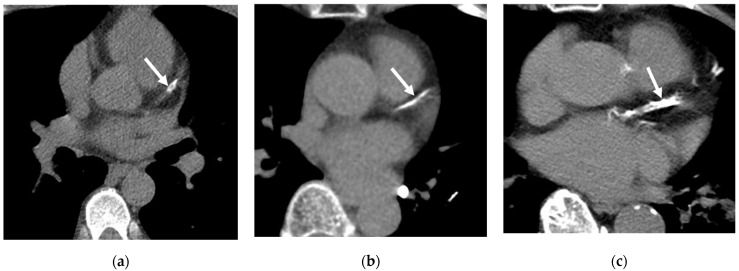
Qualitative assessment of coronary artery calcification (CAC) by low-dose computed tomography (LDCT). Axial LDCT scans demonstrate varying degrees of CAC. (a) Mild CAC with isolated flecks of calcification (arrow) in left anterior descending artery (LAD). (b) Moderate CAC with more extensive calcification (arrow) involving two segments of LAD. (c) Severe CAC with continuous, circumferential calcification (arrow) of LAD.
